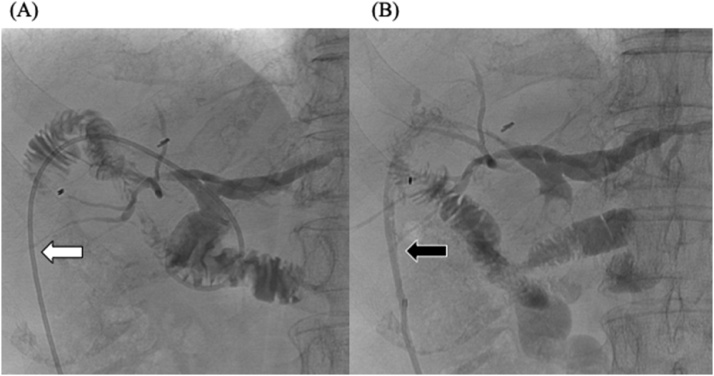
X-ray fluoroscopic examination on the 41st postoperative day. (A) The catheter was in the branch of the right intrahepatic bile duct (white arrow), and no leakage was detected. (B) The external fistula was completely formed (black arrow), and there was no abscess.
